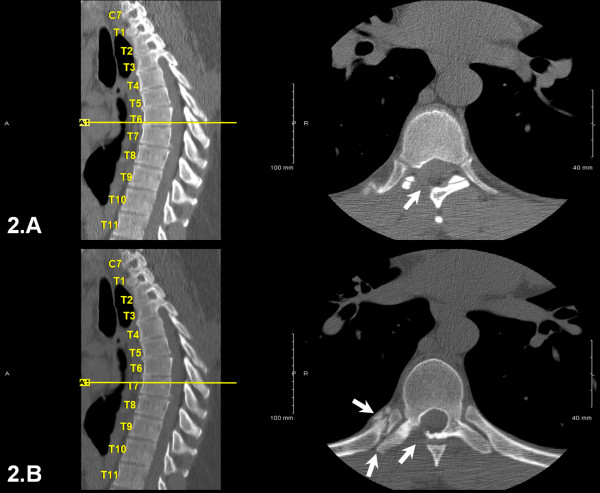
CT scans of the thoracic spine after first discectomy and decompression. A. complete right sided laminectomy at T6-7 (arrow). B. partial laminectomy at T7-8 (arrow), a fractured right T7 rib (double arrow).
